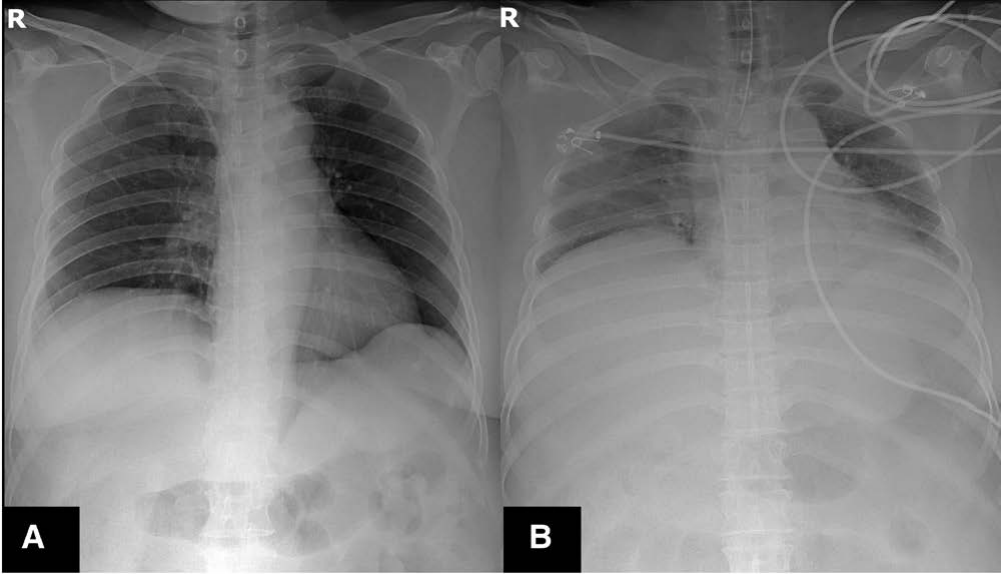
(A) Chest radiography revealing definite abdominal distension with lung volume reduction compared with (B) preoperative image.
